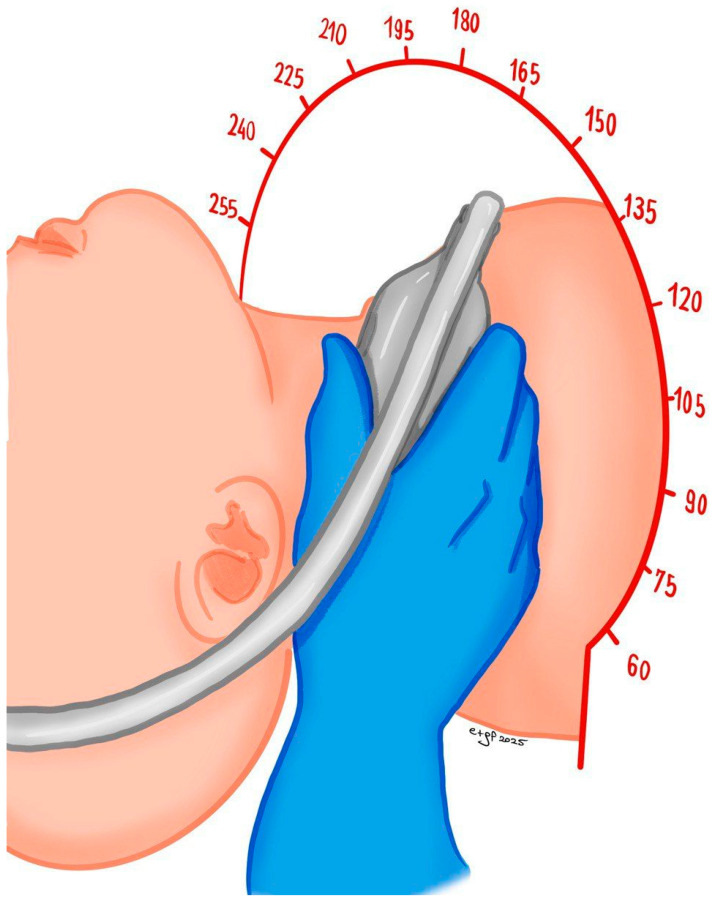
Patient and operator position for carotid ultrasound study.
